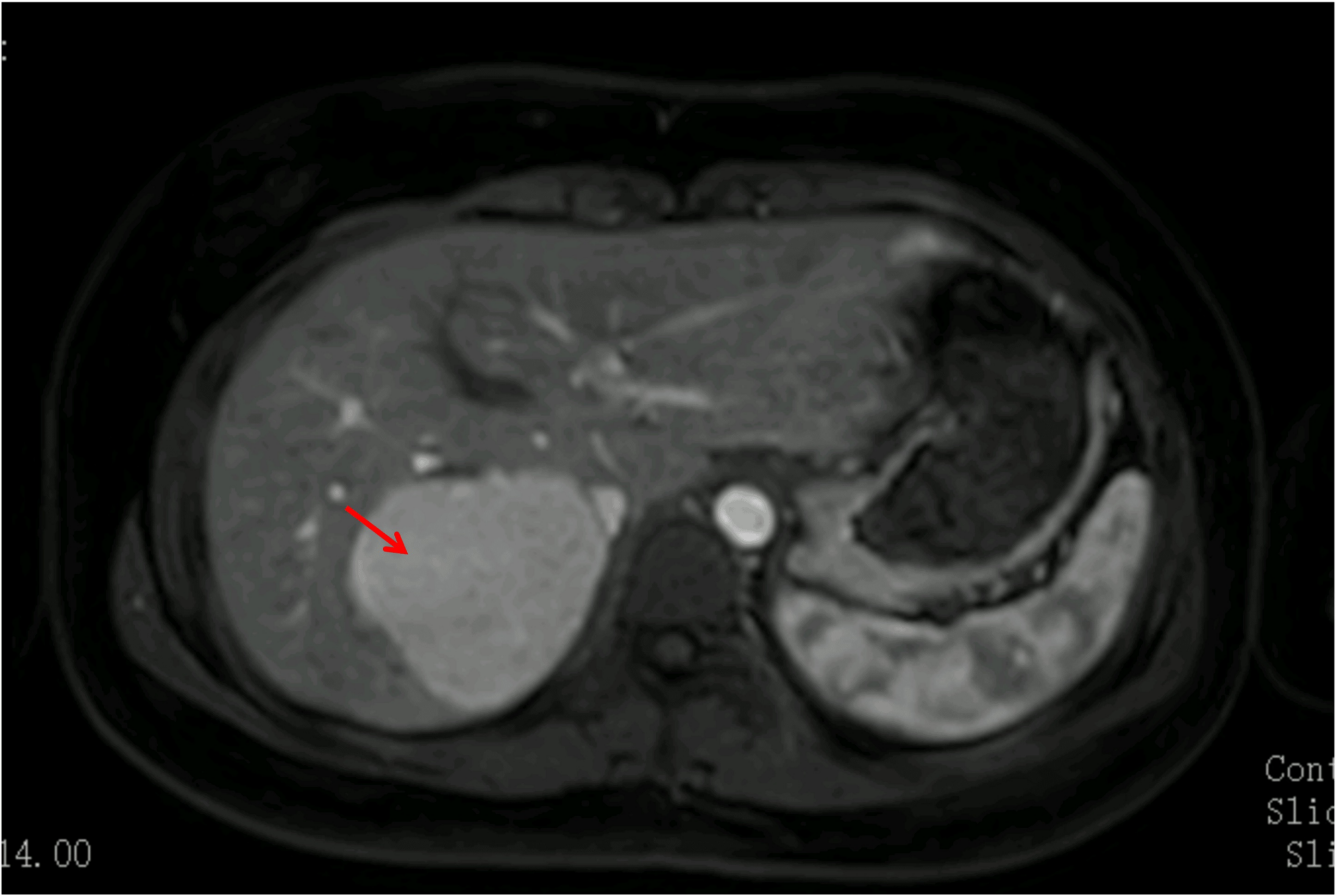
Axial abdominal MRI showing a well-circumscribed lesion in segment VII of the liver (arrow), with homogeneous arterial enhancement MRI: magnetic resonance imaging
